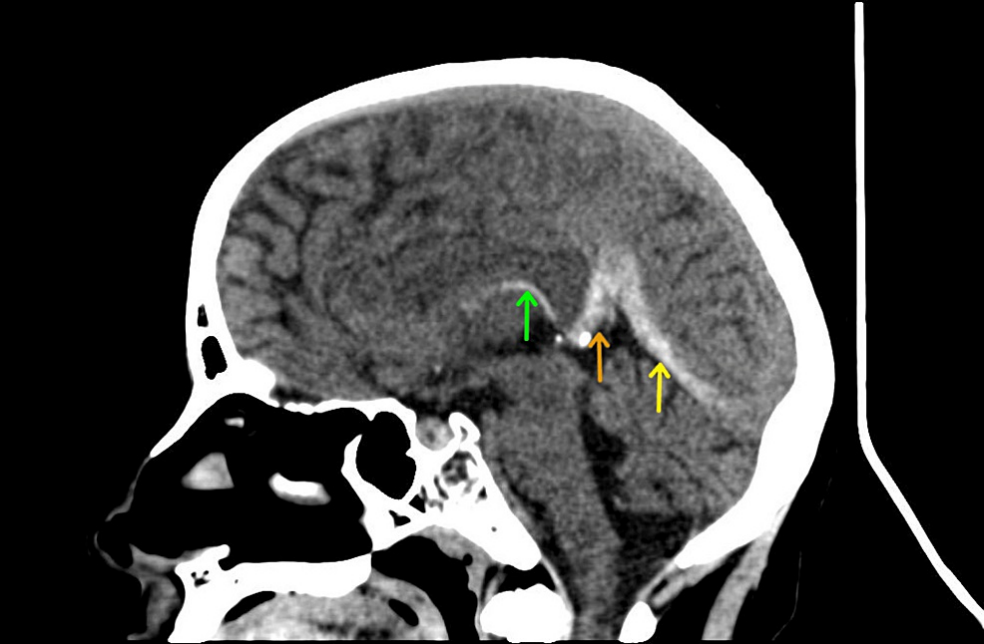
Sagittal non-contrast CT of the brain pre-treatment showing hyperdense internal cerebral vein (green arrow), the vein of Galen (orange arrow), and straight sinus (yellow arrow) consistent with cerebral venous thrombosis.
